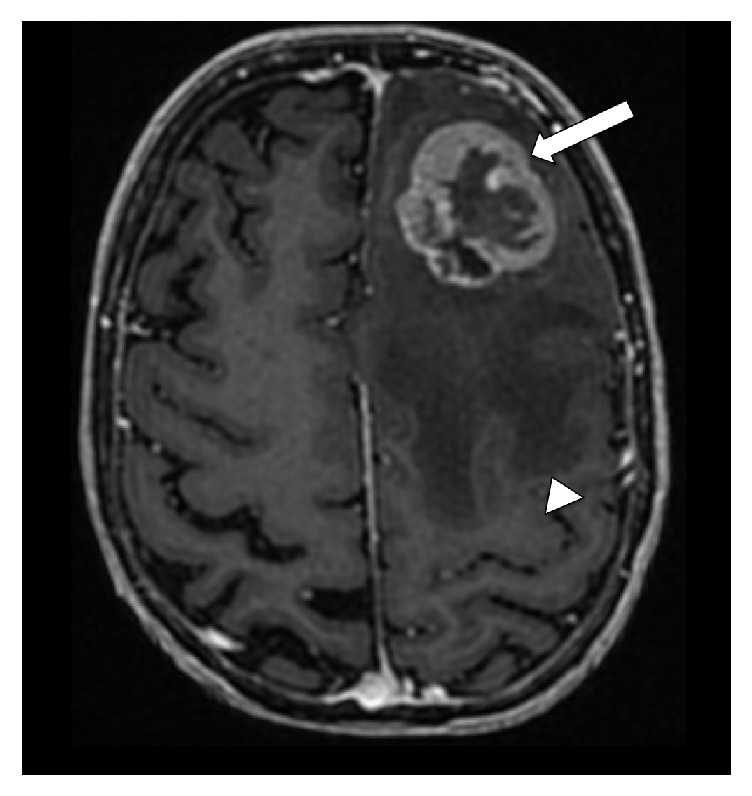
Surgical planning MRI with contrast shows an irregular left frontal mass (arrow) with surrounding edema (arrow head).
